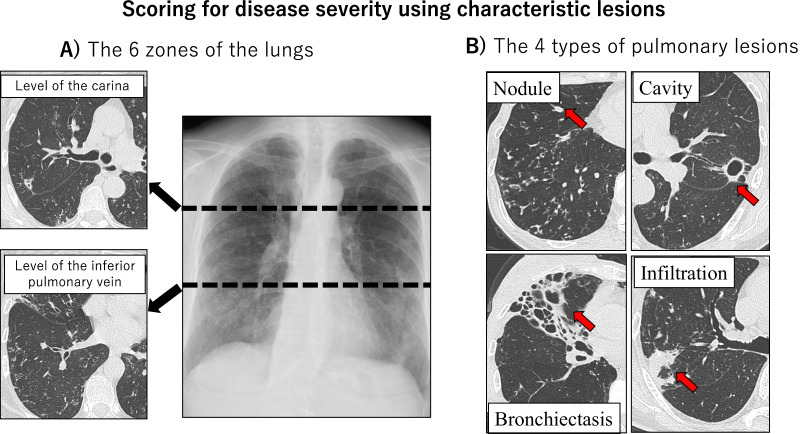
Scoring for disease severity using characteristic lesions. Representative images showing, A plain chest radiograph showing the lungs divided into six zones at the levels of the carina and inferior pulmonary vein and B the four typical lesions on HRCT described as nodule formation, cavitation, bronchiectasis, and infiltration
